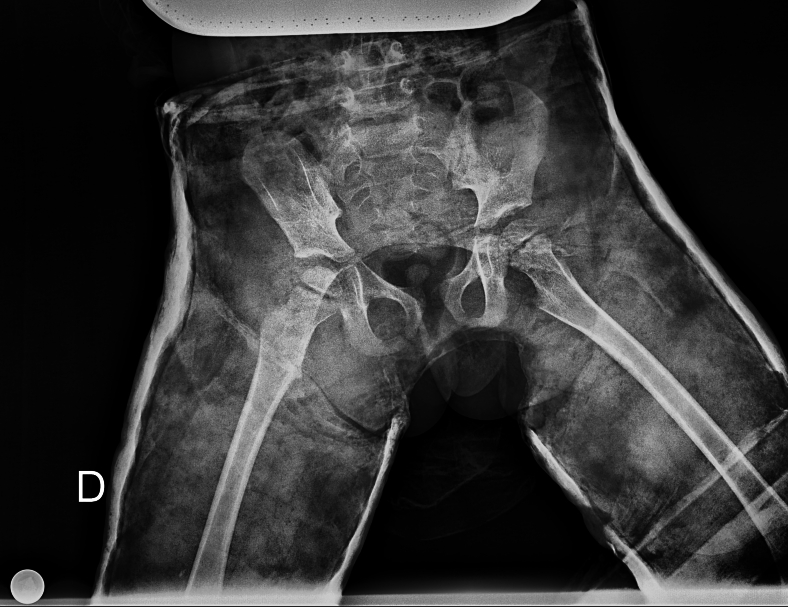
X-ray after reduction and cast application.
